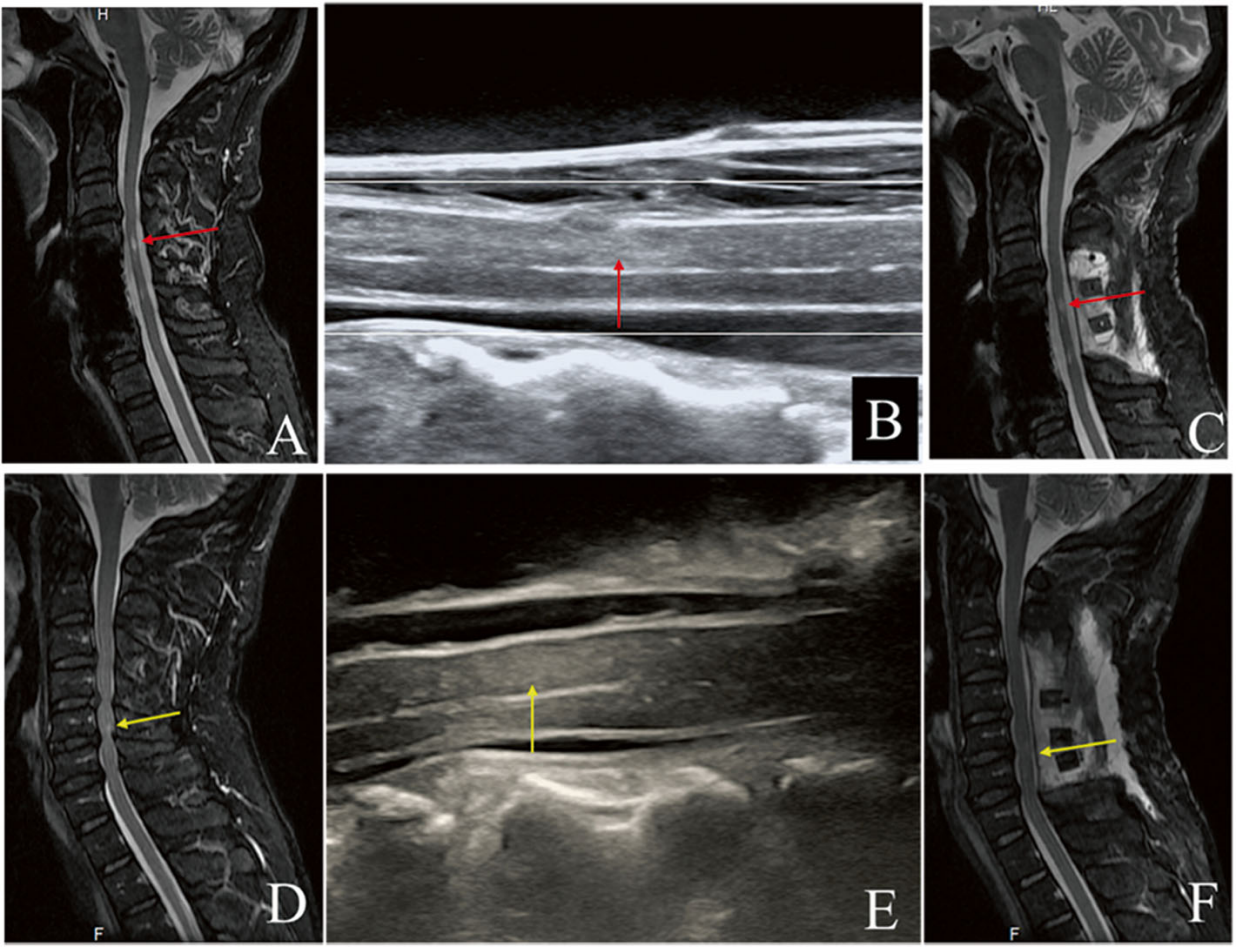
The preoperative T2W MRI, IOUSI and postoperative T2W MRI of midsagittal spinal cord of two cases (case 1: A-C, case 2: D-F). The positions of IOUSI hyperechogenicity were in line with that of preoperative T2W MRI increased signal intensity (ISI). But for the patient (case 1) with relatively low IOUSI hyperechogenicity, the postoperative ISI on T2W MRI was still existed while the patient (case 2) with high IOUSI hyperechogenicity, the postoperative ISI on T2W MRI was disappeared
